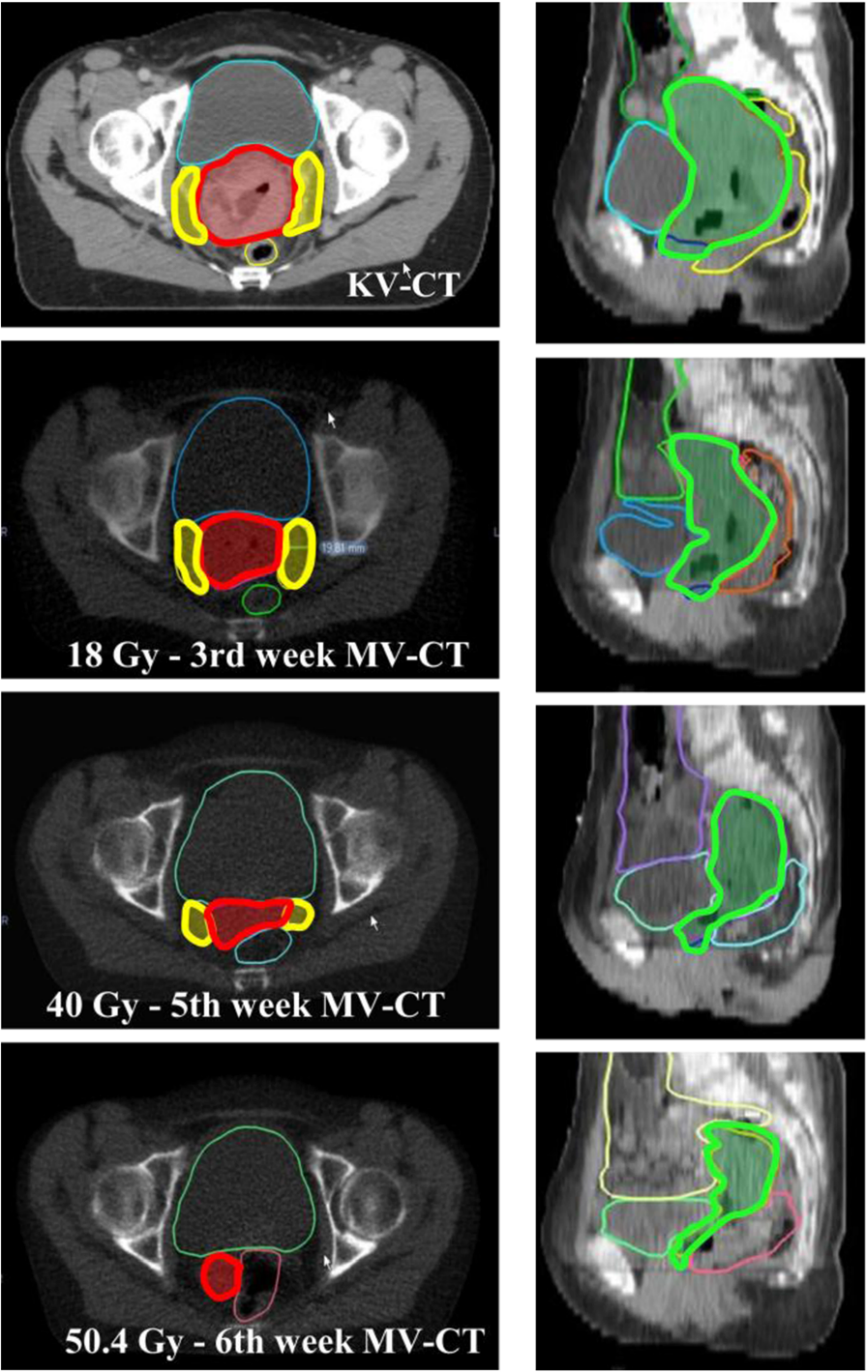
Standardized guidelines on contouring the clinical target volume - simultaneous integrated boost (CTV-SIB) on the planning-CT and weekly MV-CTs. Guidelines were adapted from Viswanathan et al. Axial and sagittal view of weekly contouring in one of the patients. The CTV-SIB structures included were: *In red*: The cervix containing the tumor. *In yellow*: Parametriums were divided as: · If inner half invasion: Butterfly shape structure <2 cm from cervix edge. · If outer half invasion: Butterfly shape structure > 2 cm from cervix edge. *In green (sagittal view)*: Corpus uteri. · If there was myometrial infiltration the entire corpus uteri was included. · If there was no myometrial infiltration, a 2-cm expansion was added from the proximal extend of the superior cervix edge or to a point at which volume expands (indicating presence of uterine tissue – where the uterine cavity appears). The vagina was contoured as a 2 cm expansion inferior to cervix edge.
